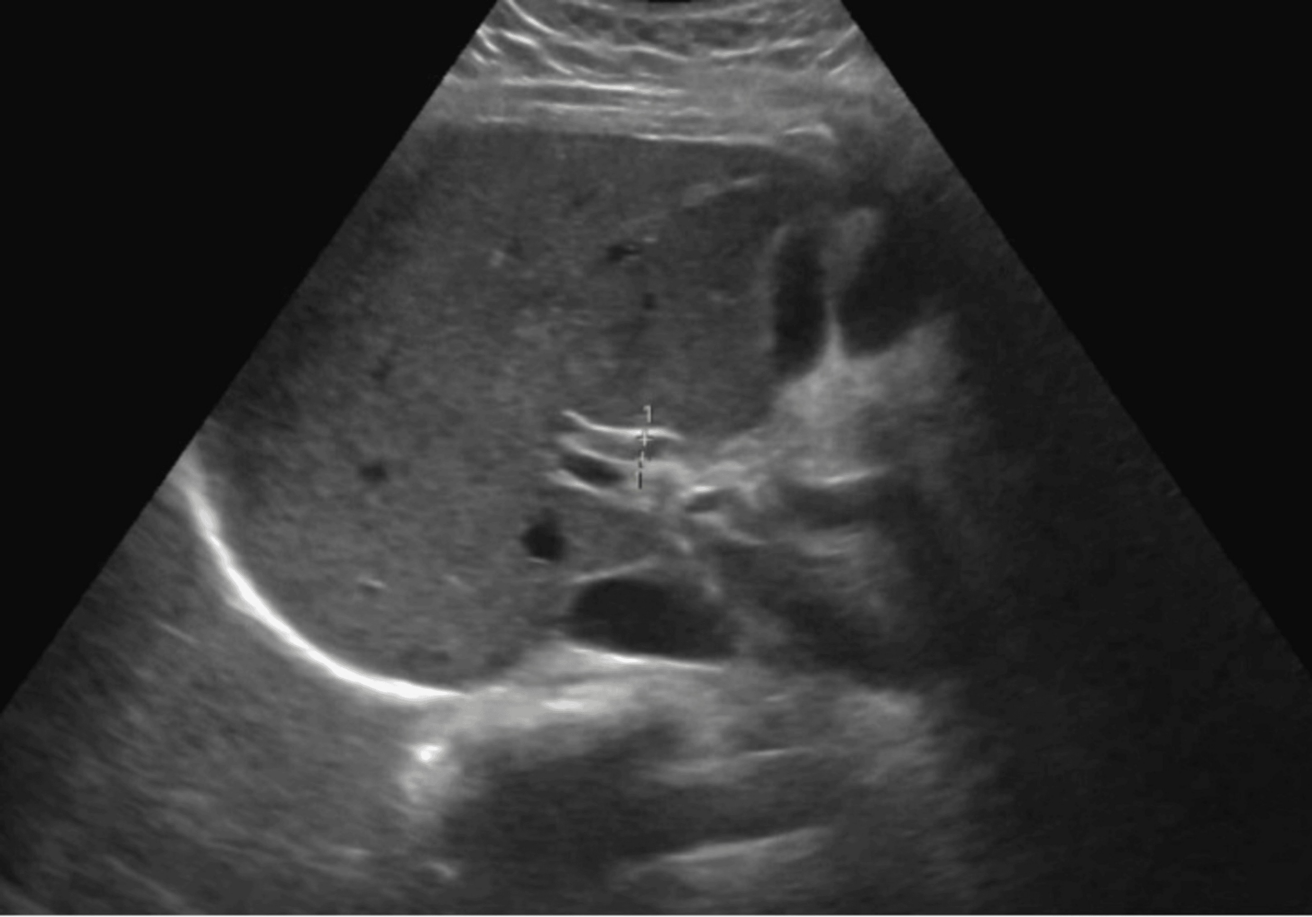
US abdomen: Questionable trace perihepatic ascites. Otherwise, unremarkable right upper quadrant sonogram.
